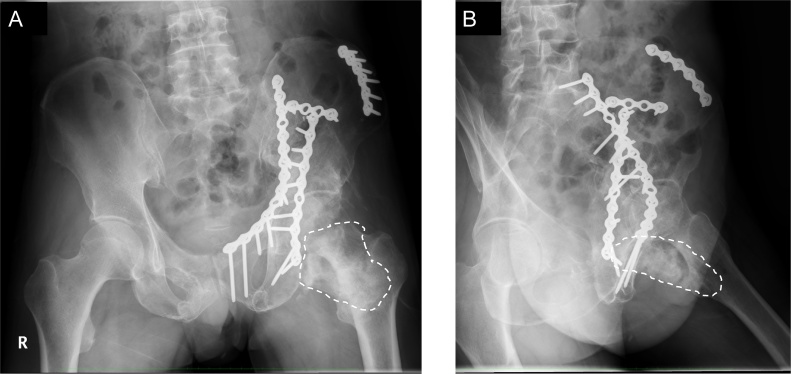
(A) Anteroposterior and (B) lateral pelvic radiographs before HO resection. HO (white dotted line) bridged the left hip posteriorly.
